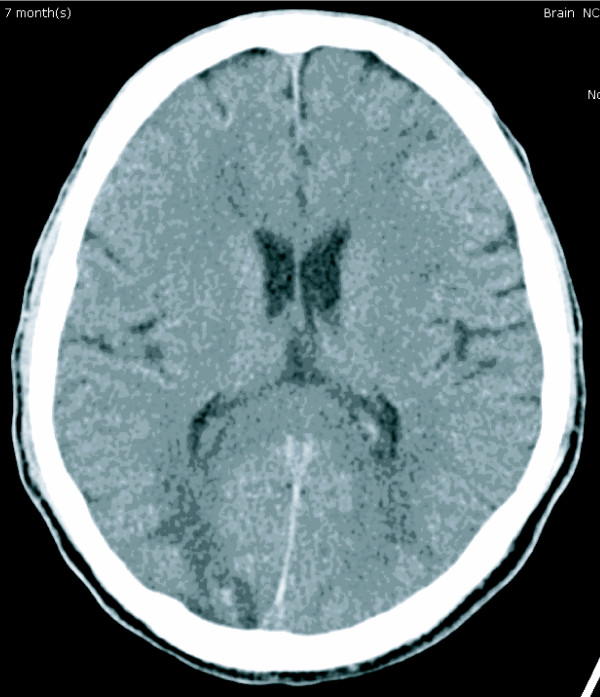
Computed tomography scan of the brain (with contrast) showing ill-defined hypodensity lesion with multiple hypodensity spots at bilateral parieto-occipito-temporal regions. The lesion was more on the right than the left cerebral hemisphere.
